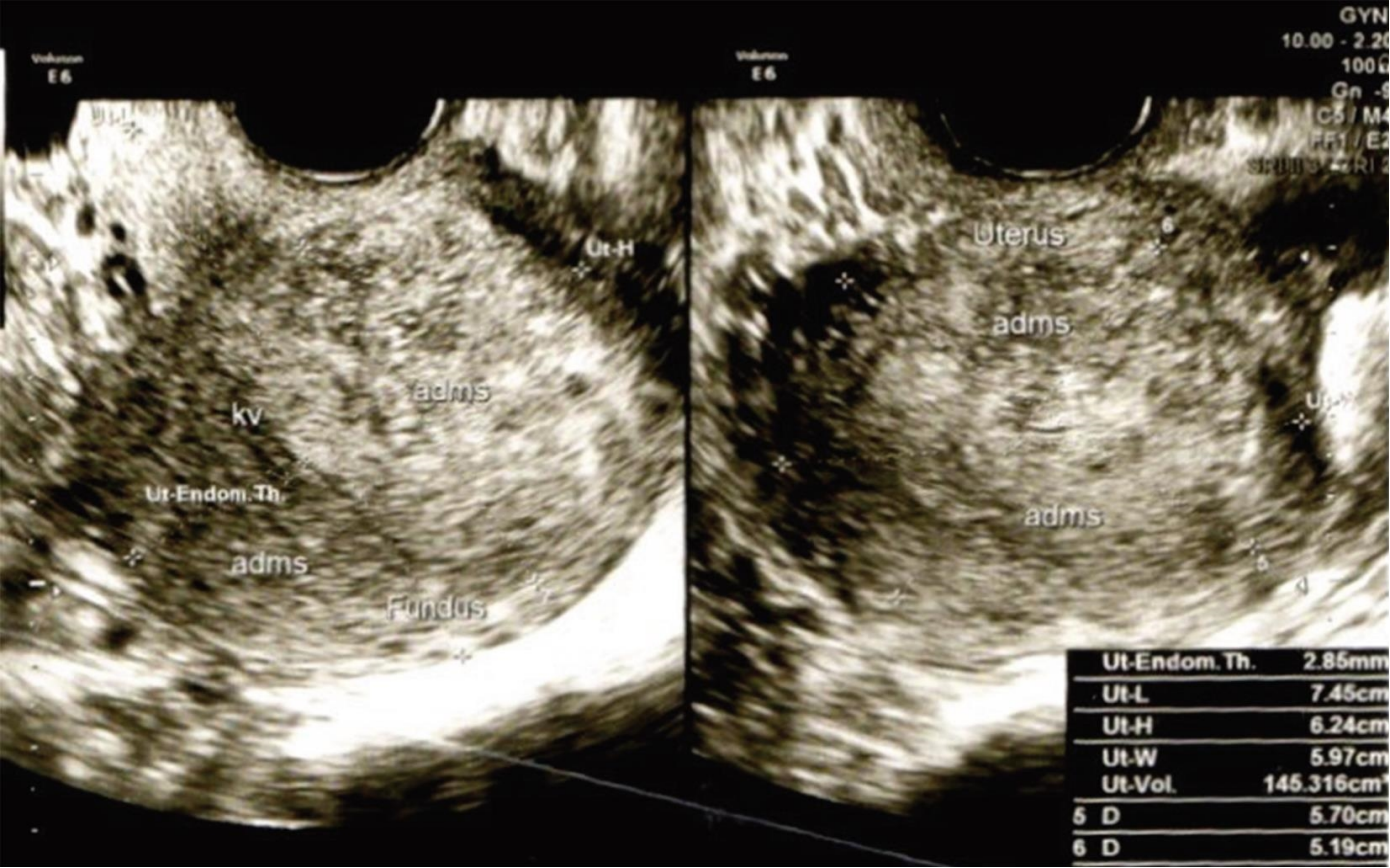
Imaging findings. Ultrasound revealed enlargement of globular shaped uterus, retroflexion with volume 145 cm^3^, not suppressing the uterine cavity EL 2.84 mm, normal cervix portio and endocervix, with no seen mass on both ovaries.
